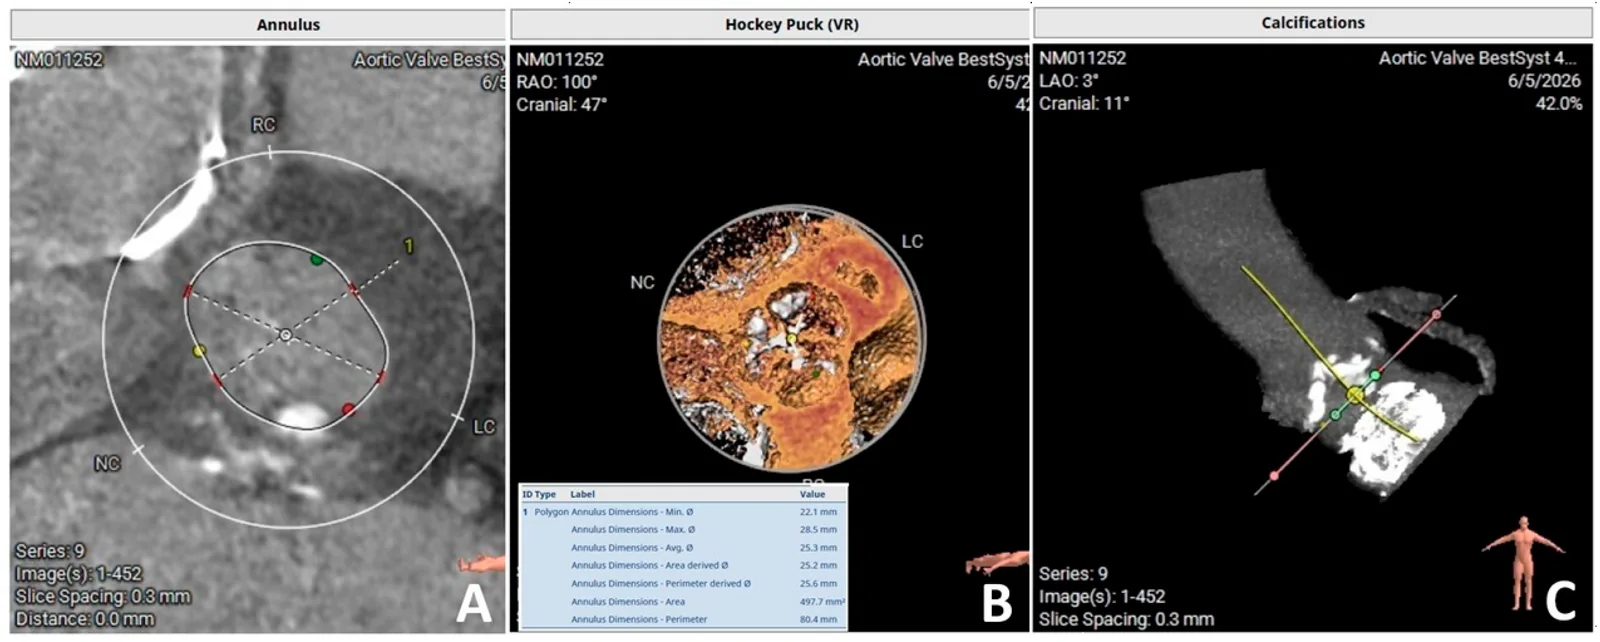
Preprocedural cardiac computed tomography—multiplanar reconstruction used for sizing and calcium assessment of the native aortic valve. (A) Annular measurements: minimum diameter 22.1 mm, maximum diameter 28.5 mm, average diameter 25.3 mm, area-derived diameter 25.2 mm, perimeter-derived diameter 25.6 mm, area 497.7 mm^2^, perimeter 80.4 mm. (B) Volume-rendered short-axis (“hockey-puck”) view of the aortic root at the level of the valve, showing the non-coronary (NC) and left coronary (LC) cusps. (C) Three-dimensional quantification of aortic valve calcification.
